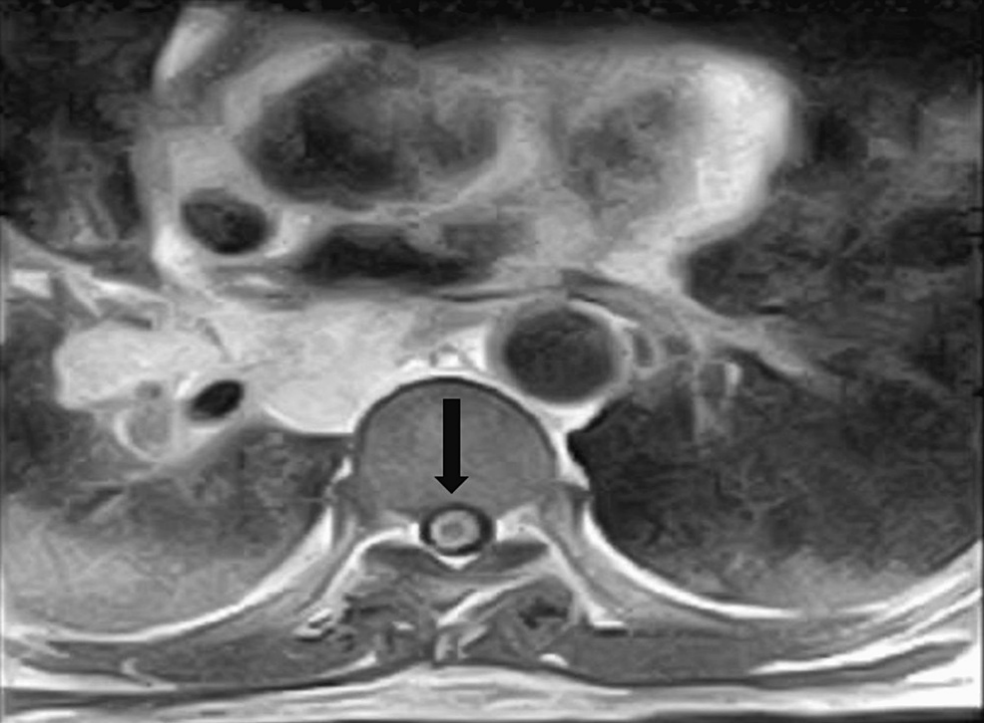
The axial T2-weighted thoracic MRI confirmed an intramedullary hypointense lesion with peripheral enhancement.
